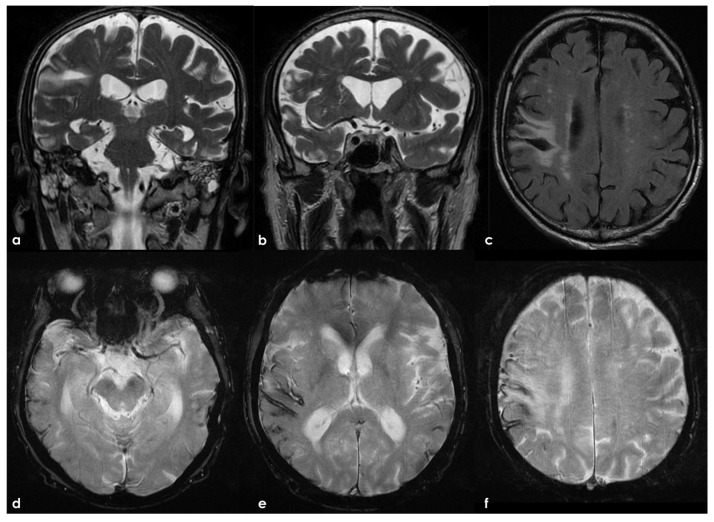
Brain MRI 6 months after the TBI, showing in T2W coronal images (panels a,b) and in FLAIR axial images (panel c) the scar of the previous contusion and a visually significant brain atrophy. Conversely, in GRE images (panels d–f), a multifocal cortical superficial siderosis emerges, mainly in the right hemisphere.
